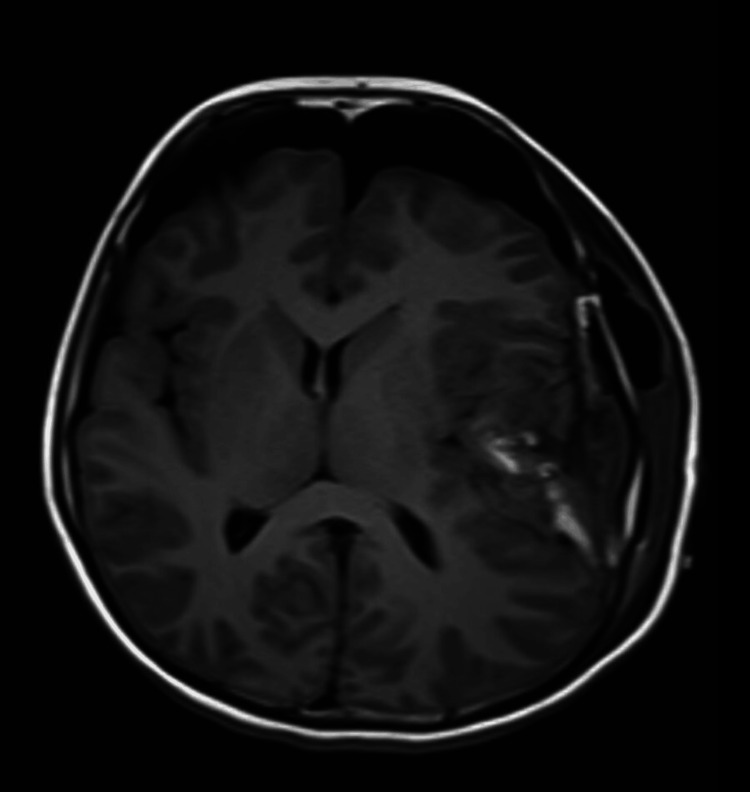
Postoperative magnetic resonance imaging scan in the T1 axial view This scan was performed three months after surgery and shows no visible enhancing lesion.
